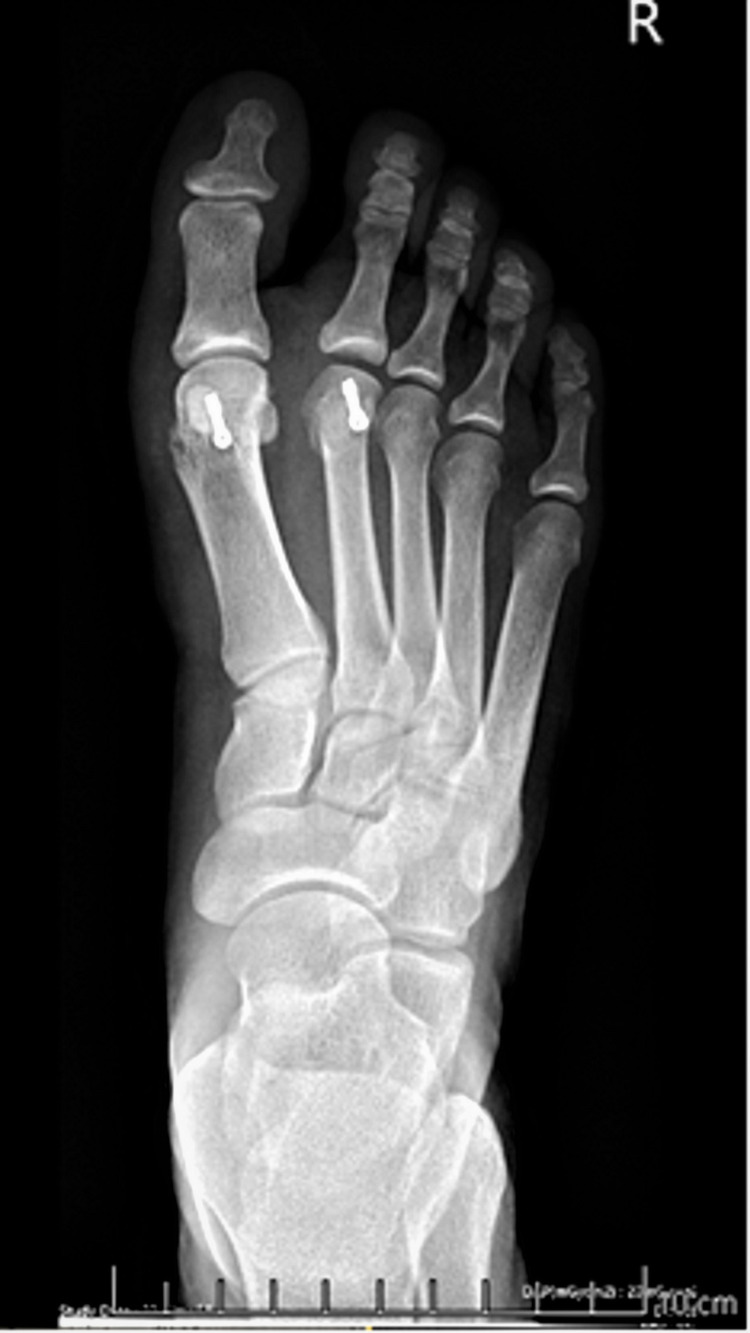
Postoperative anteroposterior weight bearing X-ray of the patient in the previous image. Two Herbert type screws were used to fix both osteotomies.
